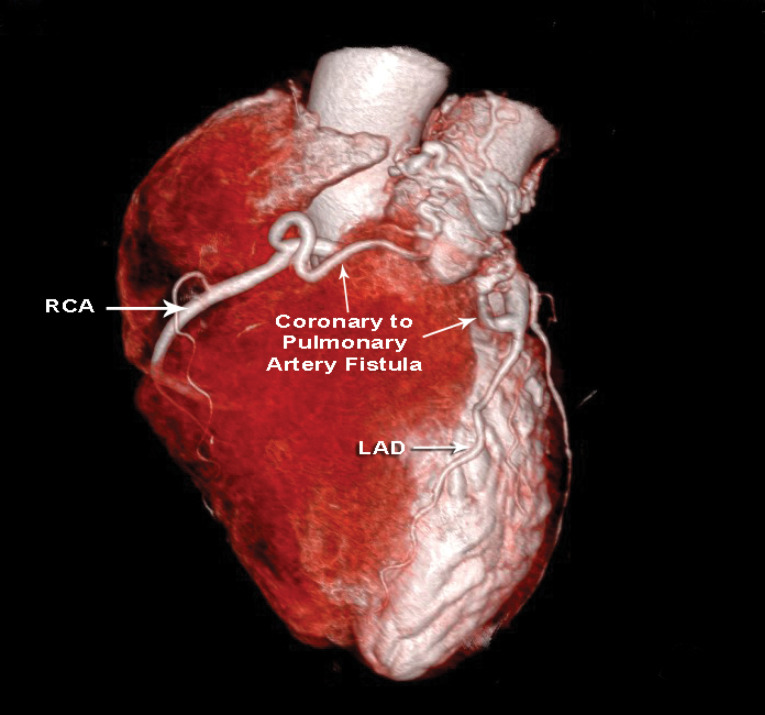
On opening up the chest there were multiple collaterals seen over the anterior surface of pulmonary (Figure 2). Patient was put on cardiopulmonary bypass using aortic and bicaval cannulation. Cross clamp was applied on the aorta to include the pulmonary artery as well to prevent the cardioplegia to run off. Using the CT Scan findings which showed the internal opening of the fistula to be on the left lateral aspect of the MPA. A 4 cm longitudinal incision was placed on the pulmonary artery. A 2 mm opening was seen on the left lateral aspect of the pulmonary artery (Figure 3). No other opening was seen. On administering cardioplegia, it was seen to drain through the opening. The opening was closed with 5-0 prolene pledegeted suture (Figure 4). The closure of fistula was tested by running the cardioplegia solution. No leakage was seen in the PA. Pulmonary artery was closed in double layer using double layer 6-0 prolene sutures. Mitral valve replacement was done using 27 mm St jude Masters mechanical valve in usual manner. Patient was weaned off bypass and shifted to recovery with good hemodynmics. Patient had an uneventful postoperative period and was discharged on the 7th post-operative day.
